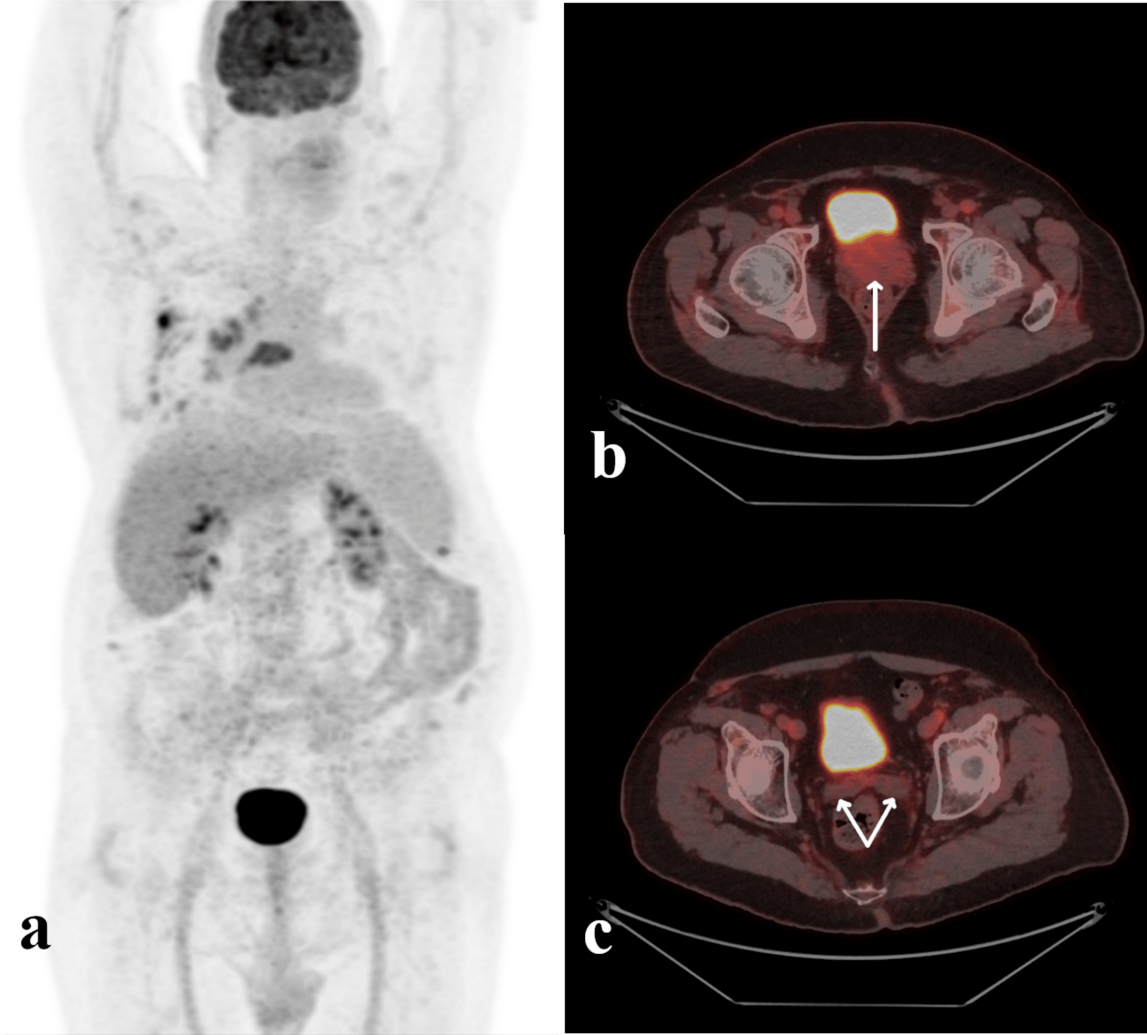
PET/CT imaging prior to radical prostatectomy (a,b,c) (a) PET scan, whole-body, coronal view. (b) PET/CT fusion images, axial view. The white arrow shows the prostate gland. (c) PET/CT fusion images, axial view. The double white arrows show the seminal vesicles.
